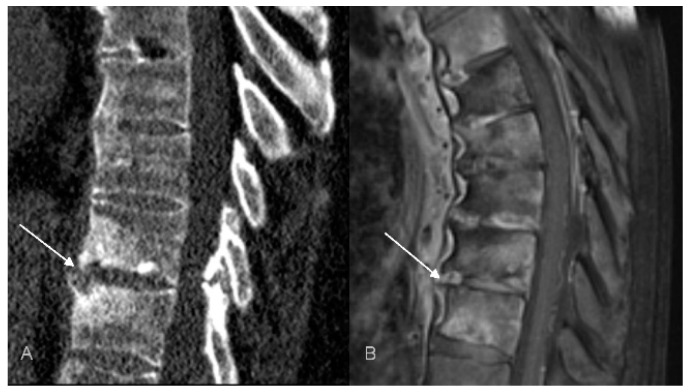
Unstable Anderson lesion in a 65-year-old patient with SAPHO syndrome. Sagittal CT reformat (A) and MRI (B) shows interruption of syndesmophytes and disc space widening (arrows).
